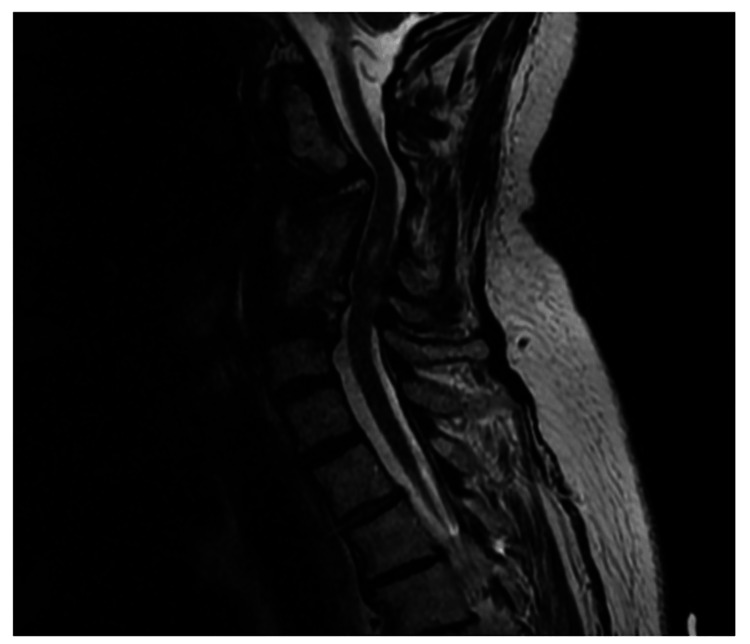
Preoperative T2 MRI showing C3-C6 cord compression with focal myelomalacia
